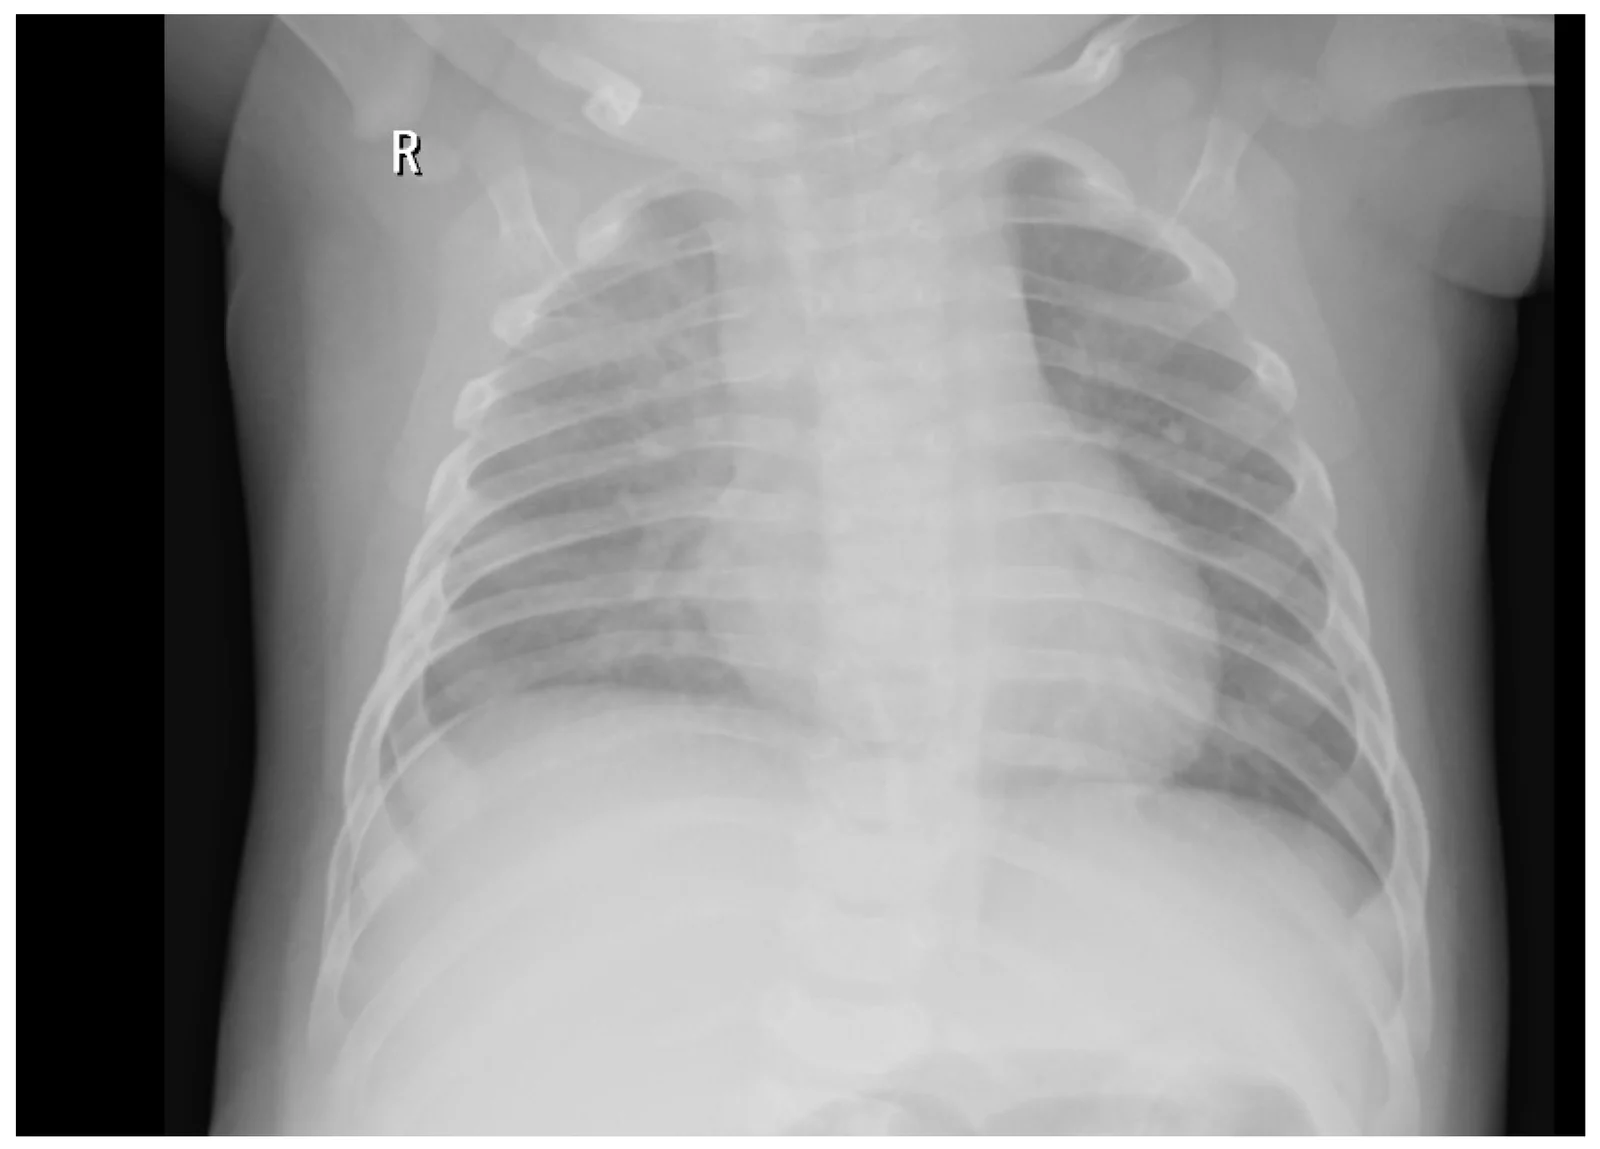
Chest X-ray showing bilateral paramediastinal interstitial infiltrates, more pronounced on the right, without consolidated foci.
